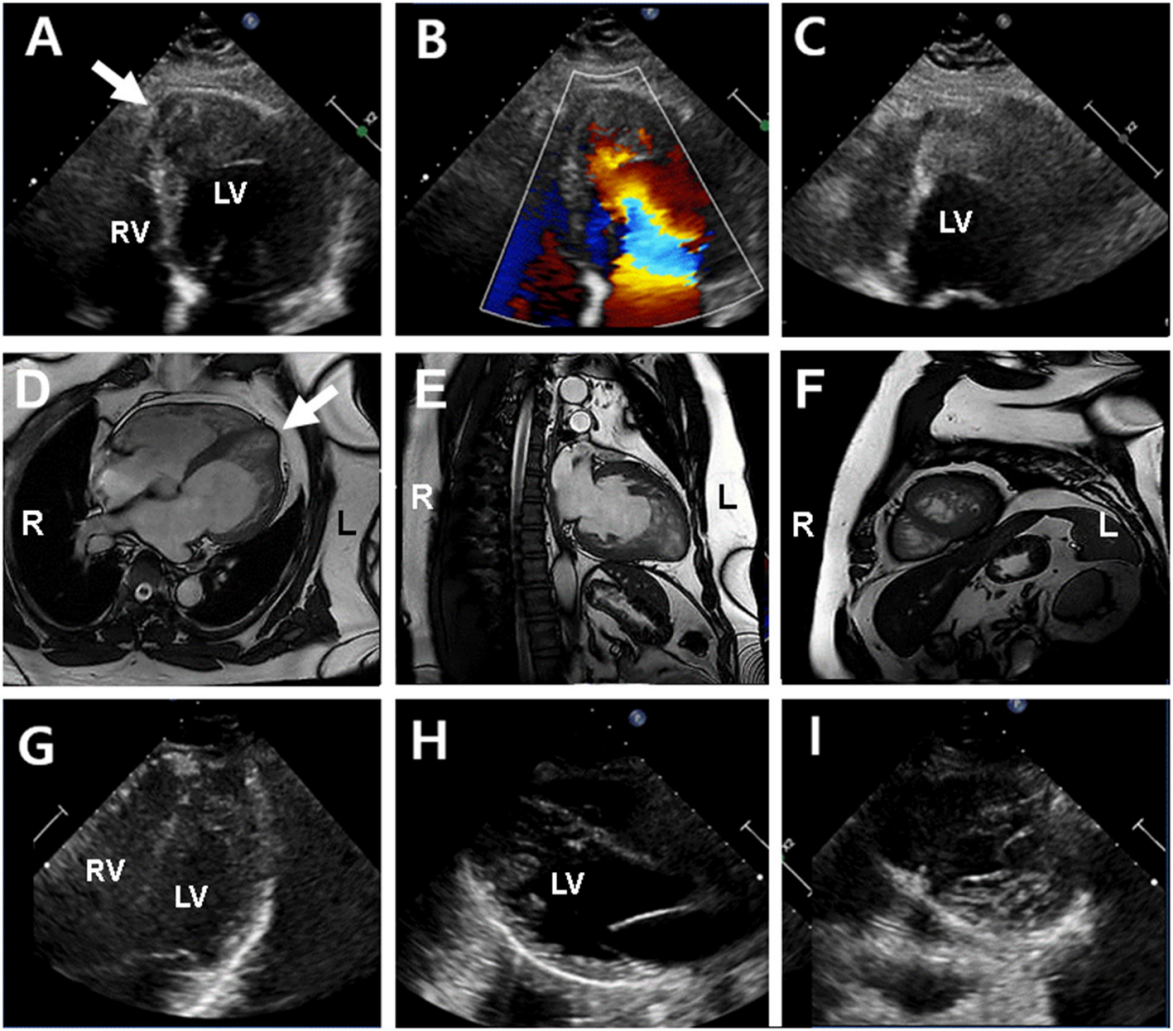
Echocardiography and cardiac magnetic resonance imaging (cMRI) in the proband's father (II-1) (upper and middle panel) and sister (III-1) (lower panel). (A–C) Echocardiography showed left ventricular hypertrophy and apical echogenic mass or dense trabeculation and apical septal aneurysm (arrow) in the proband's father. (D–F) cMRI determined dense trabeculation and focal apical septal aneurysm that considered as hypertrophic LVNC in the proband's father. (G–I) Echocardiography in proband's sister showed normal left ventricular function and geometry except for prominent trabeculation of left ventricle that diagnosed with isolated LVNC in the proband's sister.
